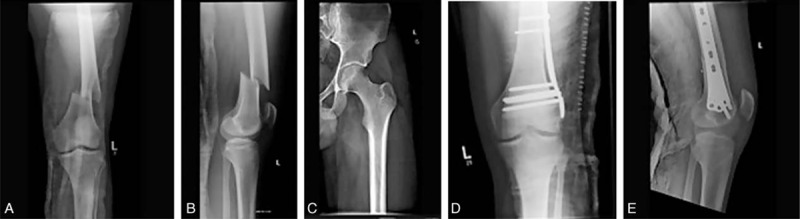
Plain film radiograph AP field preoperative (A); plain film radiograph lateral field preoperative (B); plain film radiograph of medullary canal (C); plain film radiograph AP field postoperative (D); plain film radiograph lateral field postoperative (E).
